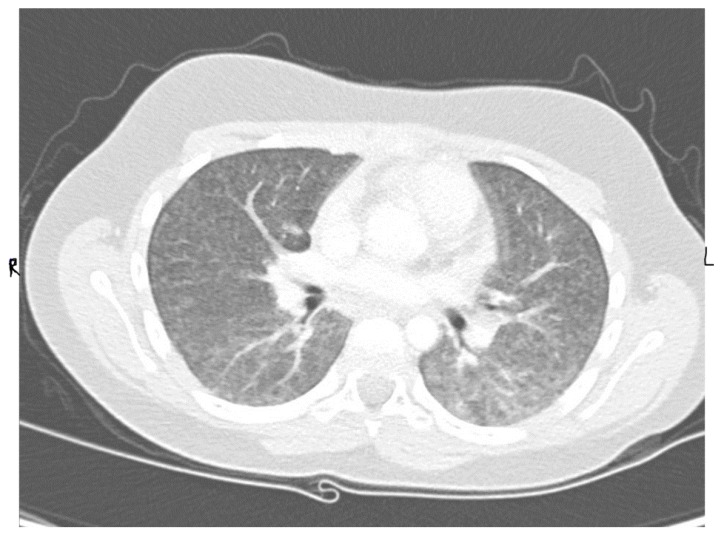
Patient CT scan of the chest showed diffusely reticular-nodular opacities in both lungs involving lung bases and the posterior segments of the right and left upper and lower lobes with irregular bronchovascular marking.
